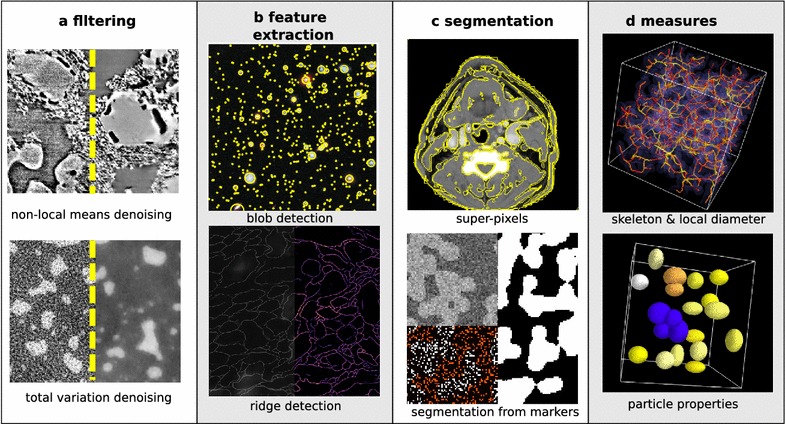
Typical image processing operations with scikit-image. Data are synthetic, unless stated otherwise. a Filtering−*Top* non-local means denoising of an image with a fine-grained texture, acquired by in situ synchrotron microtomography during glass melting [21]. *Bottom* total-variation denoising of an image with two phases, corresponding to phase-separating silicate melts observed by in situ tomography [7]. b Feature extraction−*Top* Hubble deep field (NASA, public domain), blob detection using the Laplacian of Gaussian method. *Bottom* ridge detection using the leading eigenvalue of the Hessian matrix, neuron image from CREMI challenge (https://cremi.org/data/). c Segmentation—*Top* super-pixel segmentation of a CT slice of the human head [13], using Felzenszwalb’s algorithm [18]. *Bottom* random walker segmentation (*right*) of noisy image (*top-left corner*), using histogram-determined markers (*bottom-left corner*). d Measures—*Top* visualization of local diameter (color-coded on the skeleton curve) of an interconnected phase (represented in *violet*). *Bottom* particles color-coded according to their extent
